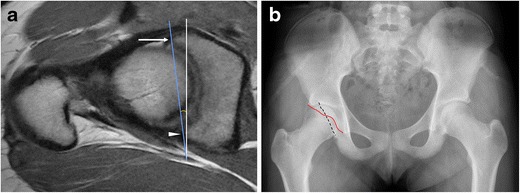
An 18-year-old girl with pincer deformity. (a) On an axial oblique PD-weighted MRI image superiorly, the anterior rim of the acetabulum (*arrow*) is located lateral to the posterior rim (*arrowhead*), indicative of superior acetabular retroversion (*blue line*). Superior acetabular retroversion of 8° is shown in this example. (b) Corresponding radiograph demonstrates the "crossover" sign where the anterior acetabular wall projects (red line) lateral to the posterior acetabular wall (black dashed line) superiorly
